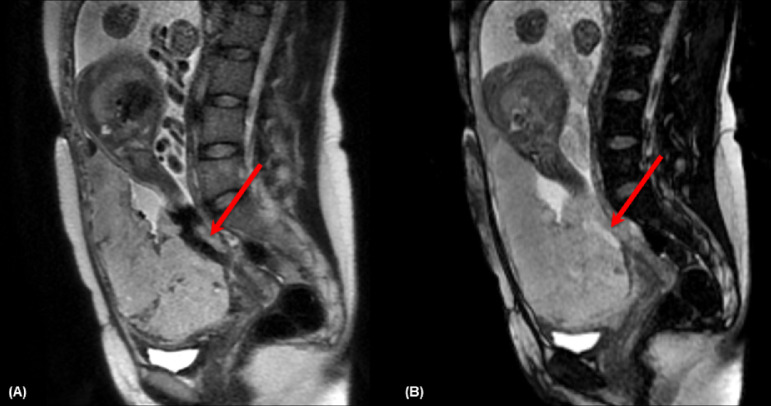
28-year-old woman at 34 weeks' gestation with two previous C-sections. (A) Sagittal T2 weighted image shows intraplacental abnormal vascularity with a flow void in the placenta (arrow), and on (B) axial FIESTA there is a high signal on the structure indicating the presence of vascular flow. - 5: > 6mm - 4: 5-6 mm - 3: 3-4 mm - 2: 1-2 mm - 1: absentIntraplacental T2 dark band: wedge-shaped areas of low signal intensity on T2-weighted images and FIESTA (19) (Fig. 3, Fig. 4).
